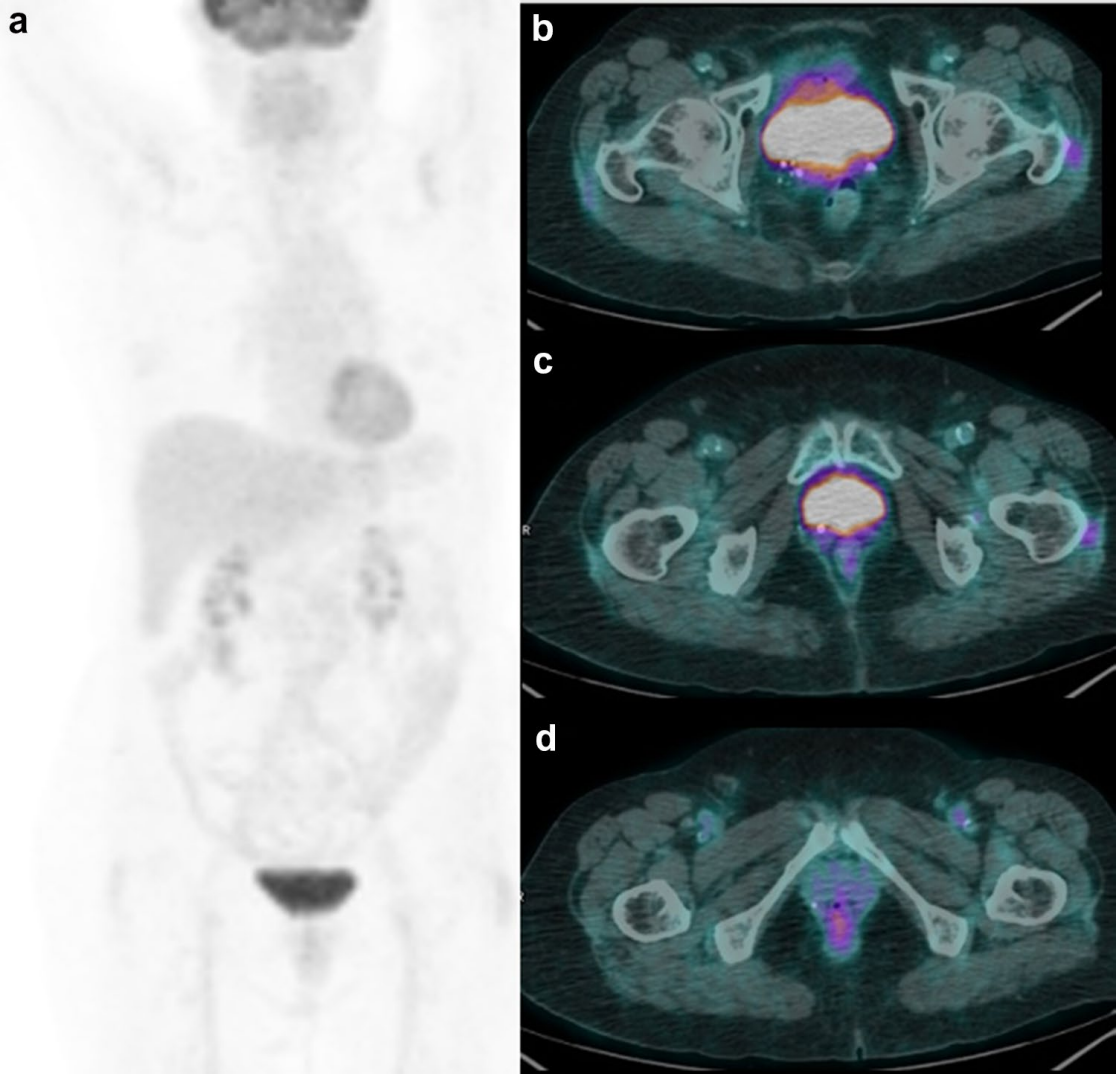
A representative case of true negative ^18^F-FDG PET/CT in a 59-year-old woman with vulvar adenocarcinoma who underwent restaging ^18^F-FDG PET/CT for suspected relapse. The recurrence was suspected by CT, but it was judged negative by PET, and confirmed to be true negative by subsequent follow-up. MIP (a) showing no increased FDG uptake in the whole body. Axial PET/CT fused images (b, c, d) detecting no pathological uptakes in the pelvis
